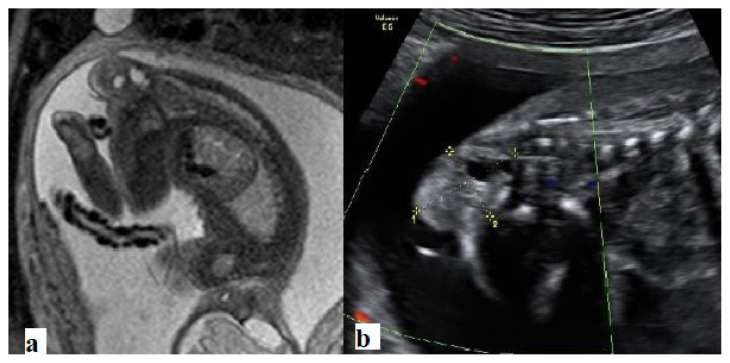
Type I sacrococcygeal teratoma. a. Sagittal T2-weighted, and b. Ultrasound images show mixed cystic and solid lesions originating from the coccyx. An intrapelvic extension is not seen.
